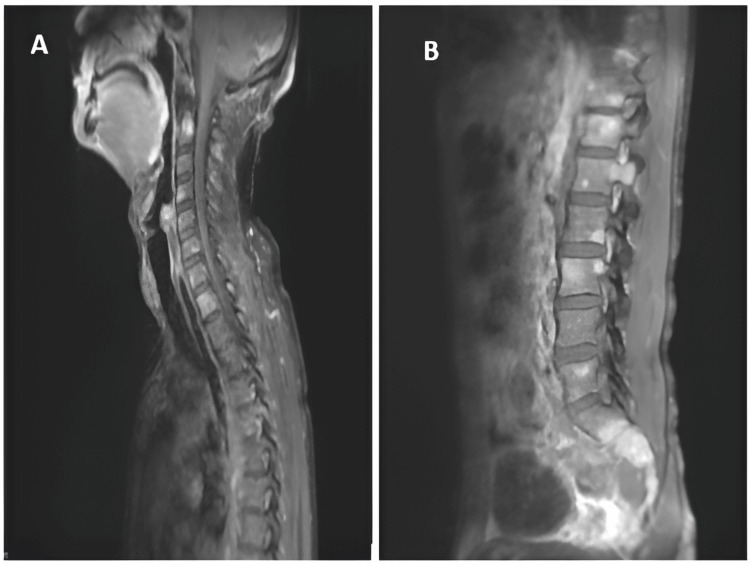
Spinal cord MRI A- Sagittal T1 acquisition with gadolinium injection showing multiple spinal lesions. B- T1 FS acquisition with gadolinium injection showing a lesion of the vertebral body from D12 and L1 to the posterior arch with epiduritis opposite.
